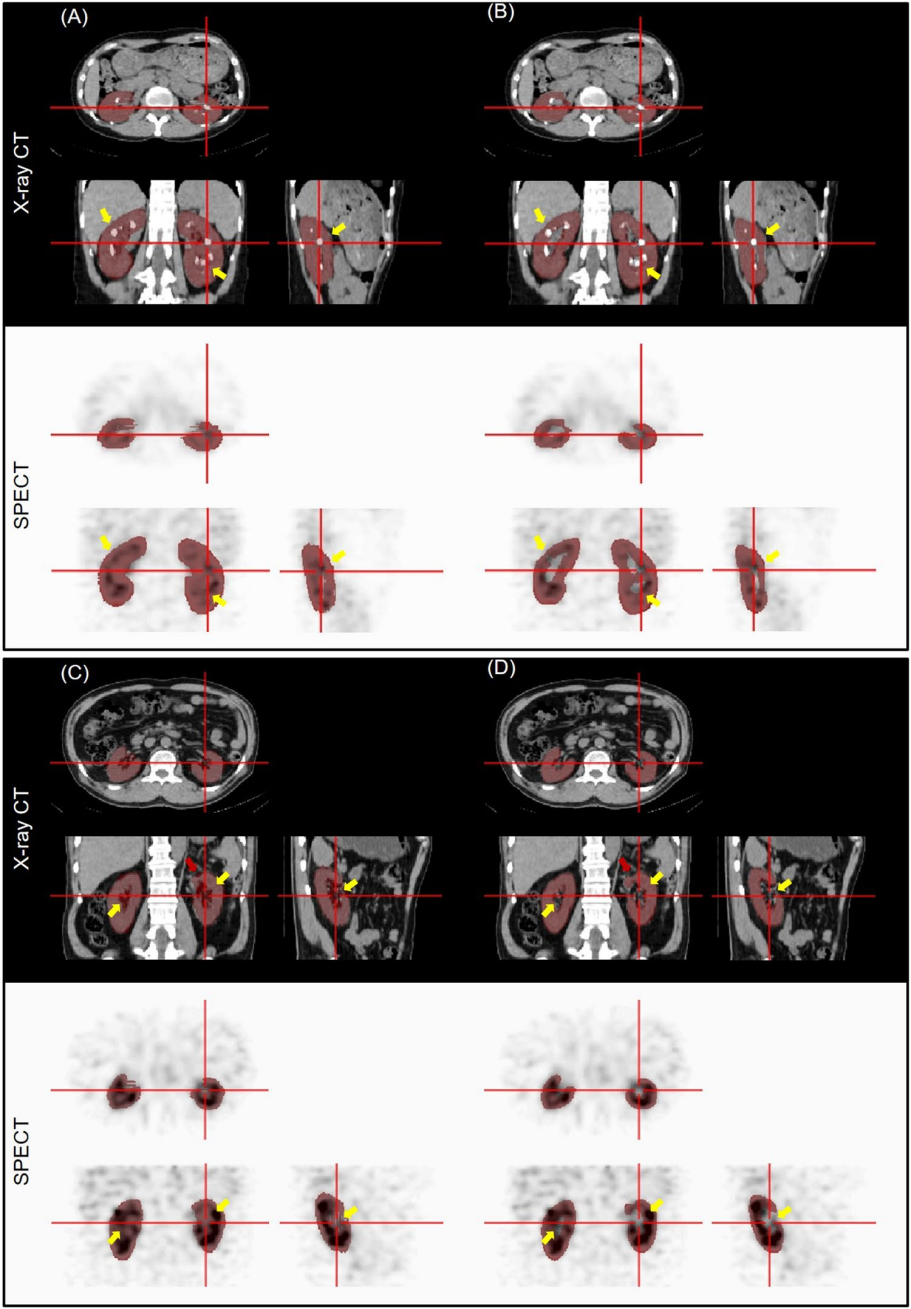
Single-photon emission computed tomography (SPECT)/computed tomography (CT) images (multiple renal stones for (A,B) and partial nephrectomy for (C,D)) and renal parenchyma volumes of interest (VOIs) for a representative test dataset (A,C) Manually segmented VOI. (B,D) Deep-learning-generated automatic VOI.
